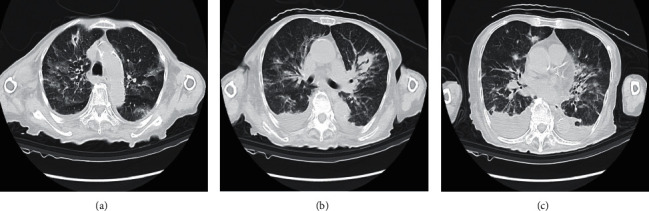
Chest CT image at the upper lobe level (a), at the carina level (b), and at the lower lobe level (c) on admission. Chest CT showed grand glass opacity in all fields with consolidation in the right and left lungs.
